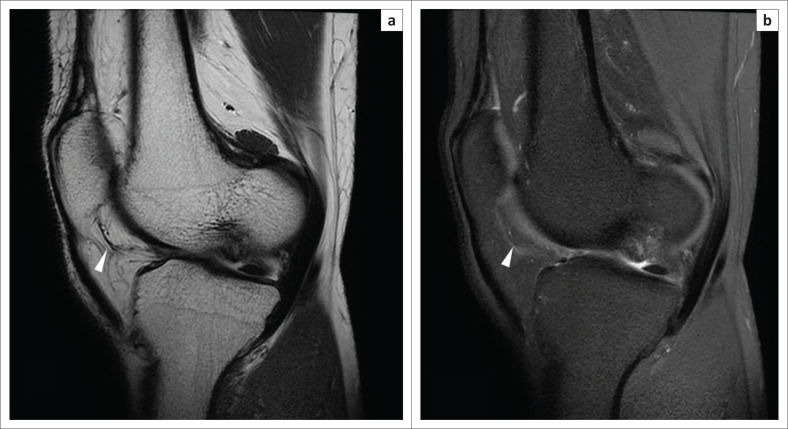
Magnetic resonance imaging of a normal infrapatellar plica: oblique sagittal T2WI (a) and oblique sagittal PDFS image (b) demonstrating the normal infrapatellar plica (arrow head) as a thin low-signal intensity structure within Hoffa’s fat pad, inserting into the inferior pole of patella.
